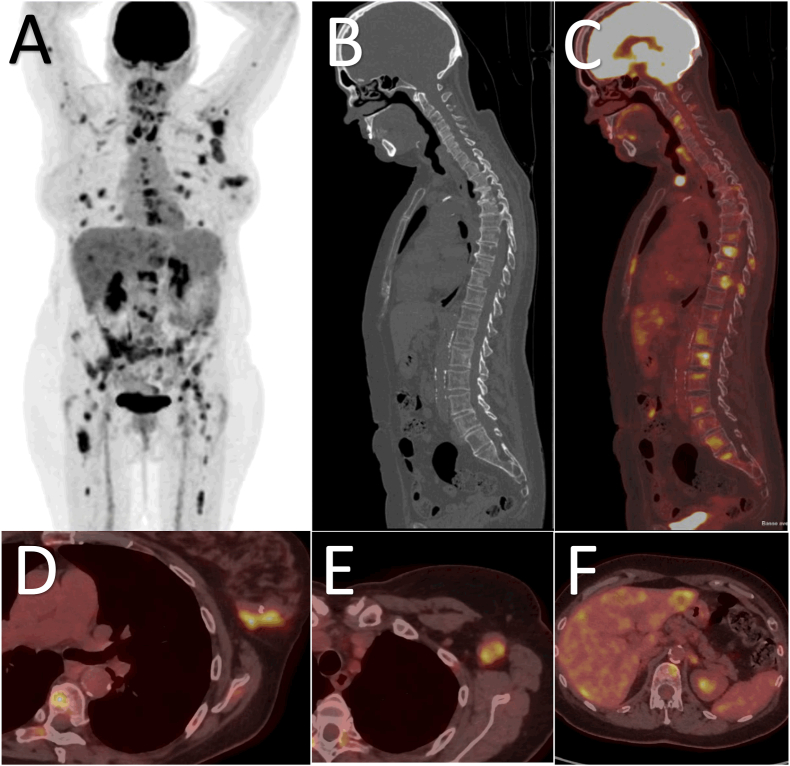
A 67-year-old woman with left NST BC, ER+, PR+, HER2- (luminal A), with clinical axillary lymph nodes was referred for primary staging with [^18^F]FDG PET/CT. MIP PET images (A) showed numerous foci of [^18^F]FDG abnormal uptake. Sagittal view (B and C, CT and PET/CT fusion images) showed numerous bone osteolytic hypermetabolic metastases. Axial PET/CT fusion images showed the primary left breast cancer (D), axillary lymph nodes (E) and liver metastases (F). The disease was classified stage IV.
